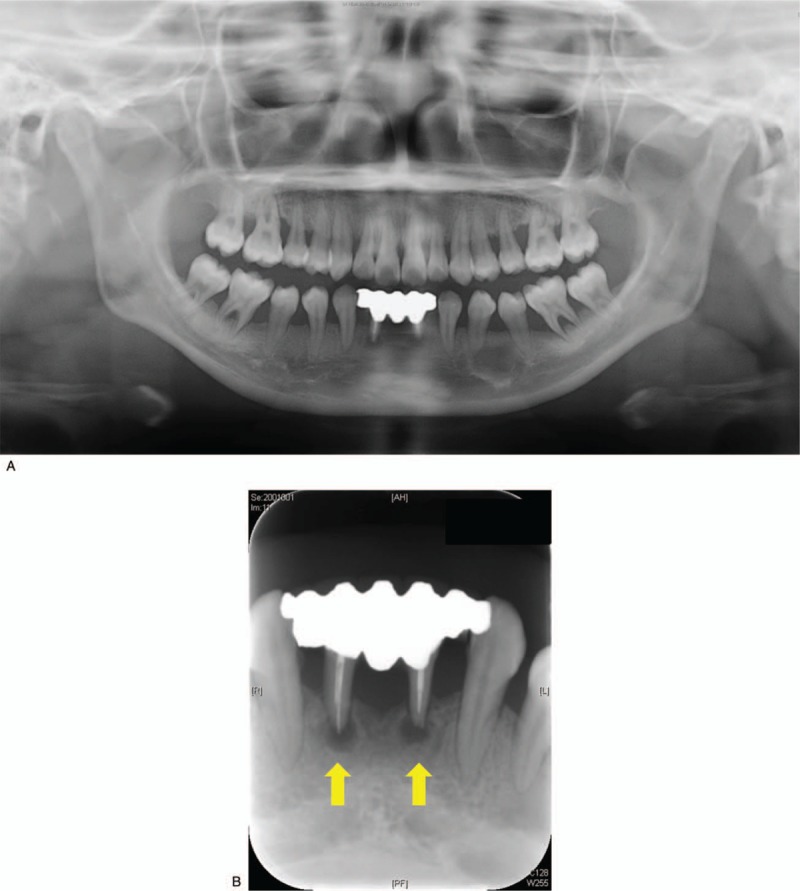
(A) Panoramic radiograph showing extreme resorption of alveolar bone. (B) Dental radiograph demonstrating apical lesions in teeth #32 and #42 (yellow arrows).
